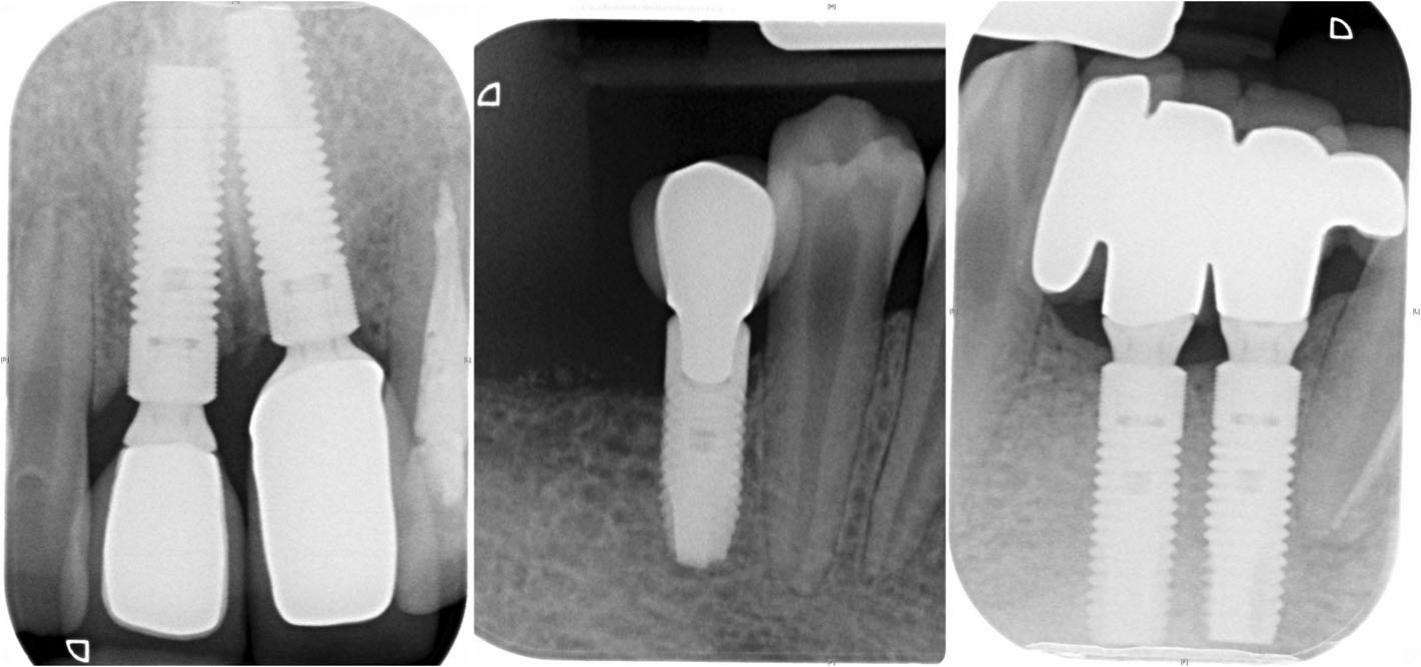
Follow-up radiographs. 11, 31 and 41 position fixtures 123 months, 21 position fixture 80 months and 46 position fixture 21 months since placement
